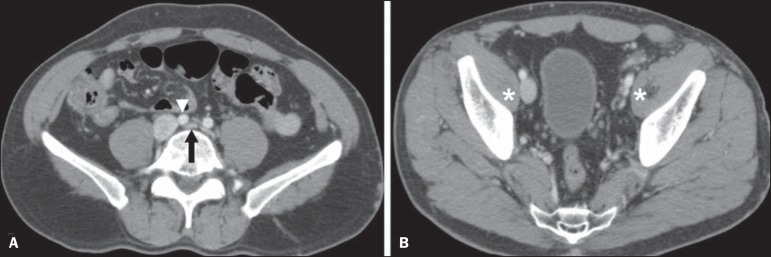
Cockett syndrome or May-Thurner syndrome. Axial CT. A:
